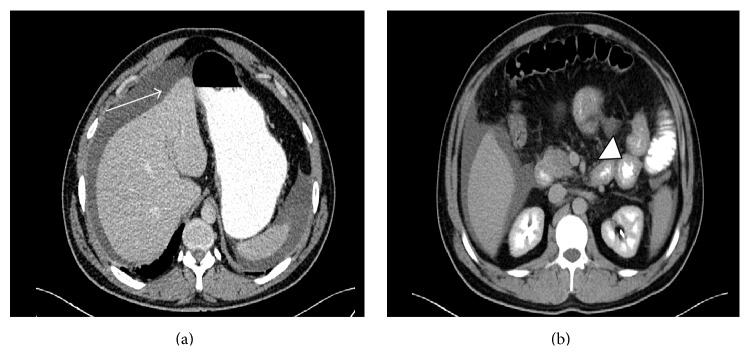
(a) Abdominal CT with oral and intravenous contrast demonstrated ascites in perisplenic and perihepatic area (white arrow). (b) Image study shows multiple segment of small bowel wall thickening (white arrow head).
